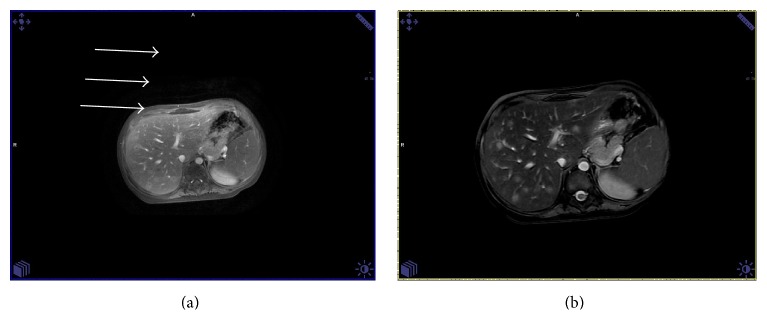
Focal liver lesions in MR imaging (hypointensive in T1-weighted contrast enhanced and fat saturated sequences (a); hyperintense in T2-weighted scans (b)).
